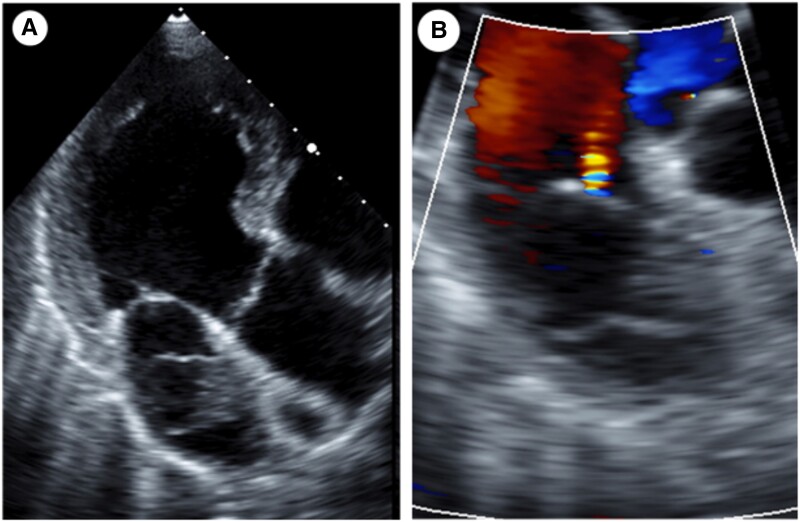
Transthoracic echocardiography showed a mobile tumour attached to the left atrial septum (*A*). A single jet was observed from the cystic lesion (*B*).
